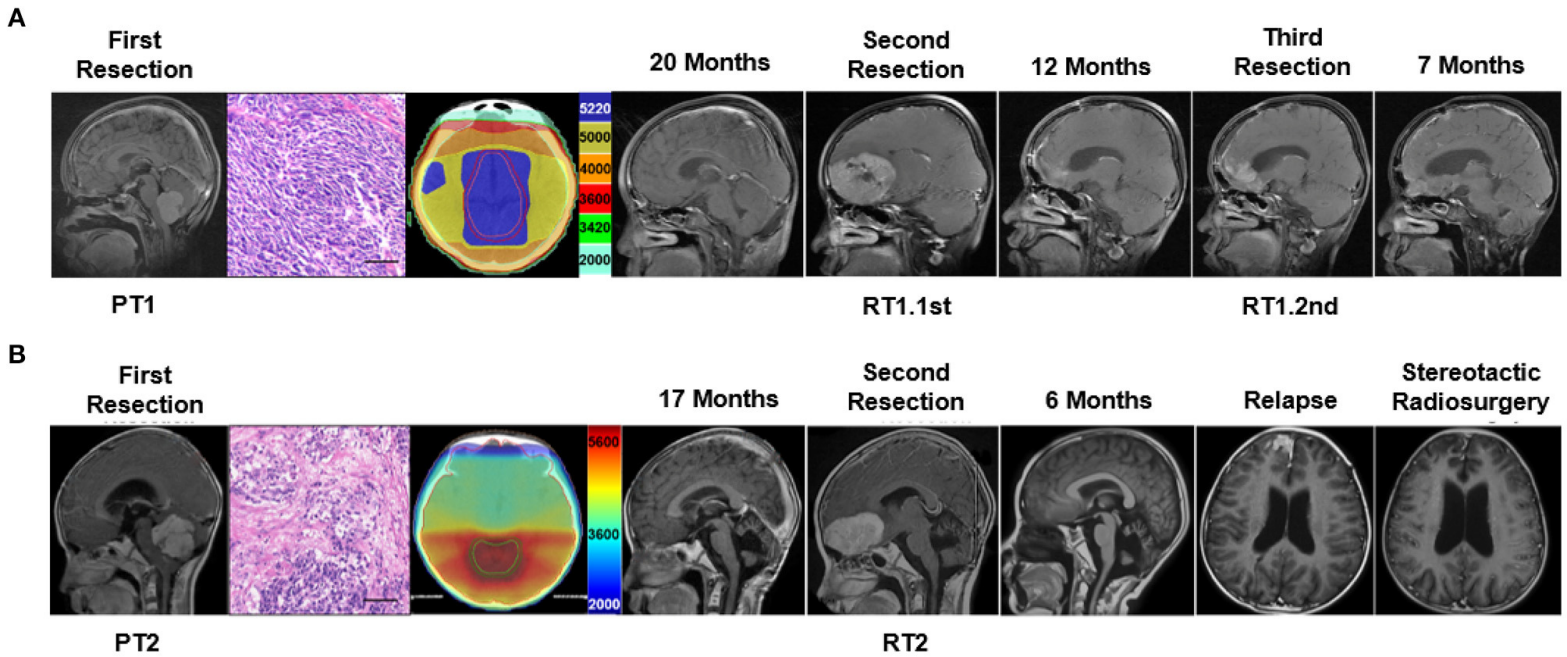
Clinical characteristic and the treatment flow process of the two cases subjected to tumor molecular profiling. (A) Delineation of radiotherapy target and MRI imaging of treatment process of case 1 and (B) case 2. Scale bar = 50 μm.
